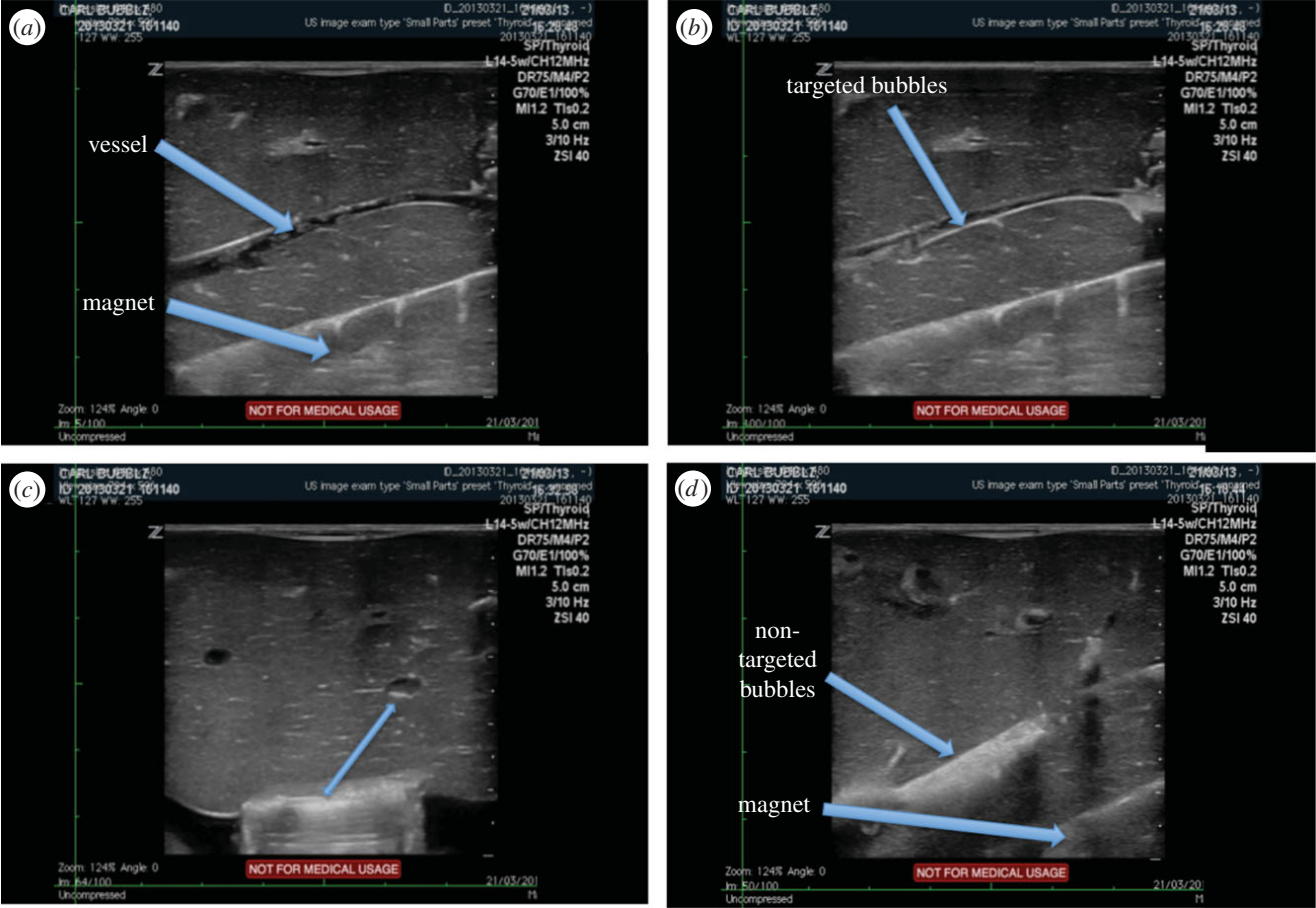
Ultrasound images showing a blood vessel in an *ex vivo* perfused liver model with a magnetic Halbach array positioned underneath it. (*a*) Before injection of magnetic microbubbles, (*b*) showing magnetic microbubbles retained at the vessel wall, (*c*) transverse view showing misalignment of the magnetic array and (*d*) non-magnetic microbubbles flowing through the vessel (second liver).
